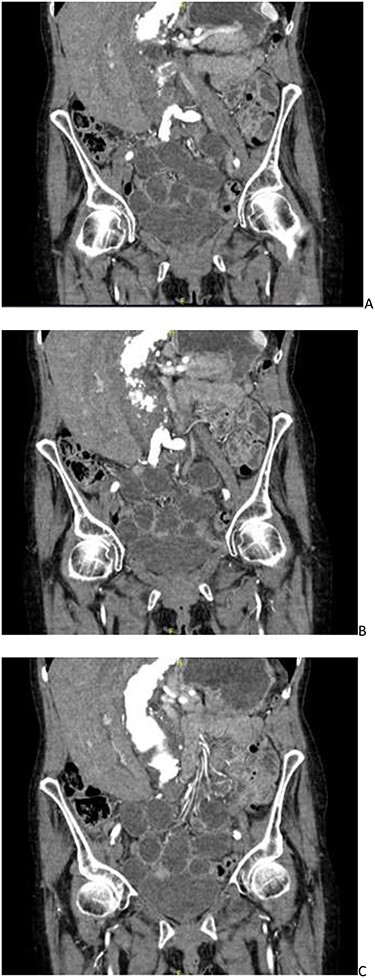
CT angiogram showing a left-sided obturator hernia; (A–C) progressing posteriorly in a coronal plane.
